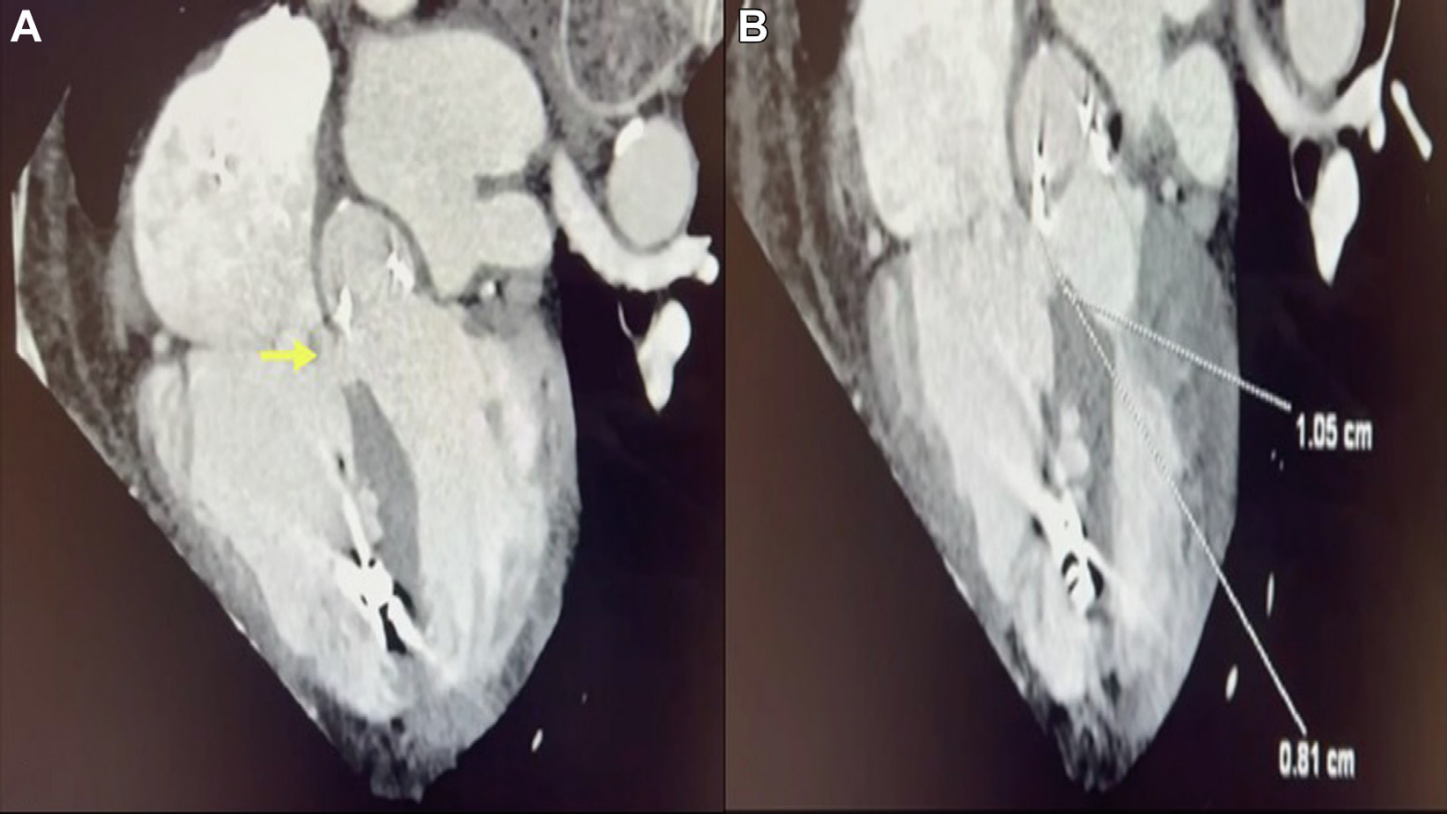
Cardiac CT Scan Cardiac CT showing the ventricular septal defect (A) and the measurements (B). CT = computed tomography.
